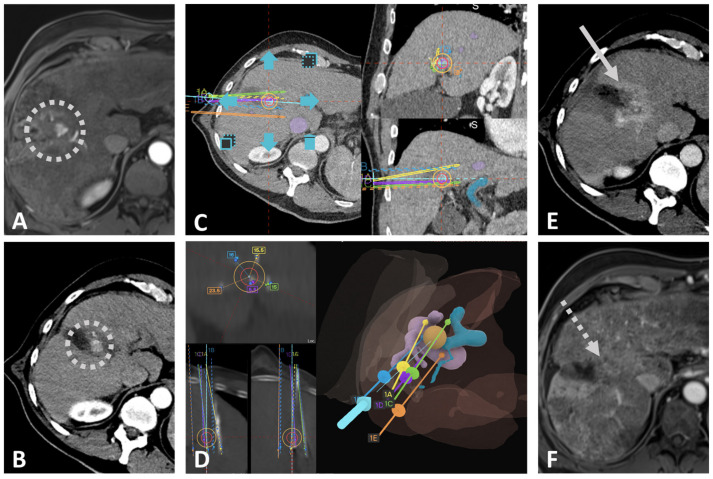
SpECT of a 64-year-old male patient with a 26 mm large iCCA located centrally in Liver Segment IVa/VIII. (A) MRI-scan in the arterial phase prior to the SpECT procedure. (B) CT-scan in the arterial phase at the start of the SpECT procedure shows the hypervascularized tumor located centrally in liver segments IVa and VIII (dotted circles) with close proximity to the right hepatic artery and the central right bile duct. (C) Planning of the trajectories for five ECT-electrodes using multiplanar reconstructions on the stereotactic navigation device, as described before [47,53]. The portal vein and the inferior vena cava including the hepatic veins were segmented in blue and purple, respectively. (D) Native control scan fused with the arterial planning scan after stereotactic insertion of the five ECT-electrodes verifies the correct placement of all five electrodes with only minimal deviations of less than two millimeters from the planned trajectories. The 3D model shows the proximity of the target lesion to the portal (blue) and hepatic (purple) veins. (E) CT scan in the arterial phase at the end of the SpECT procedure shows complete devascularization of the tumor and the directly adjacent liver tissue with a hyperemic rim around the ablation zone (solid arrow). (F) MRI-scan in arterial phase three months after the SpECT procedure shows PTE (dotted arrow indicates the former site of the tumor) without residual vital tumor or LTP.
